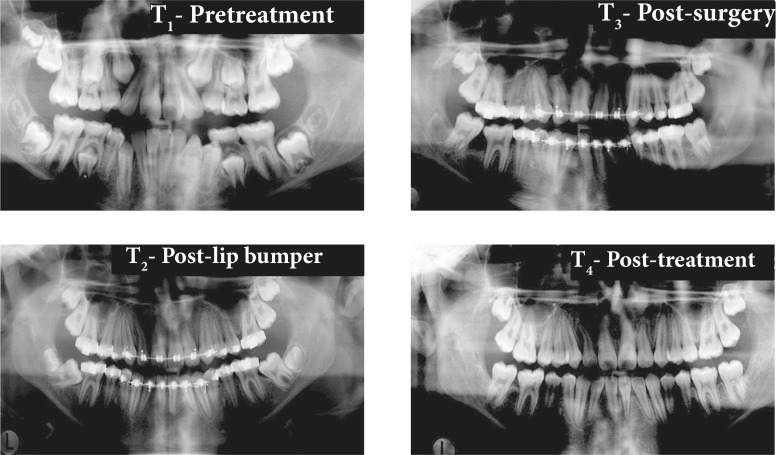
Panoramic radiographs of one of the patients who had second molar
